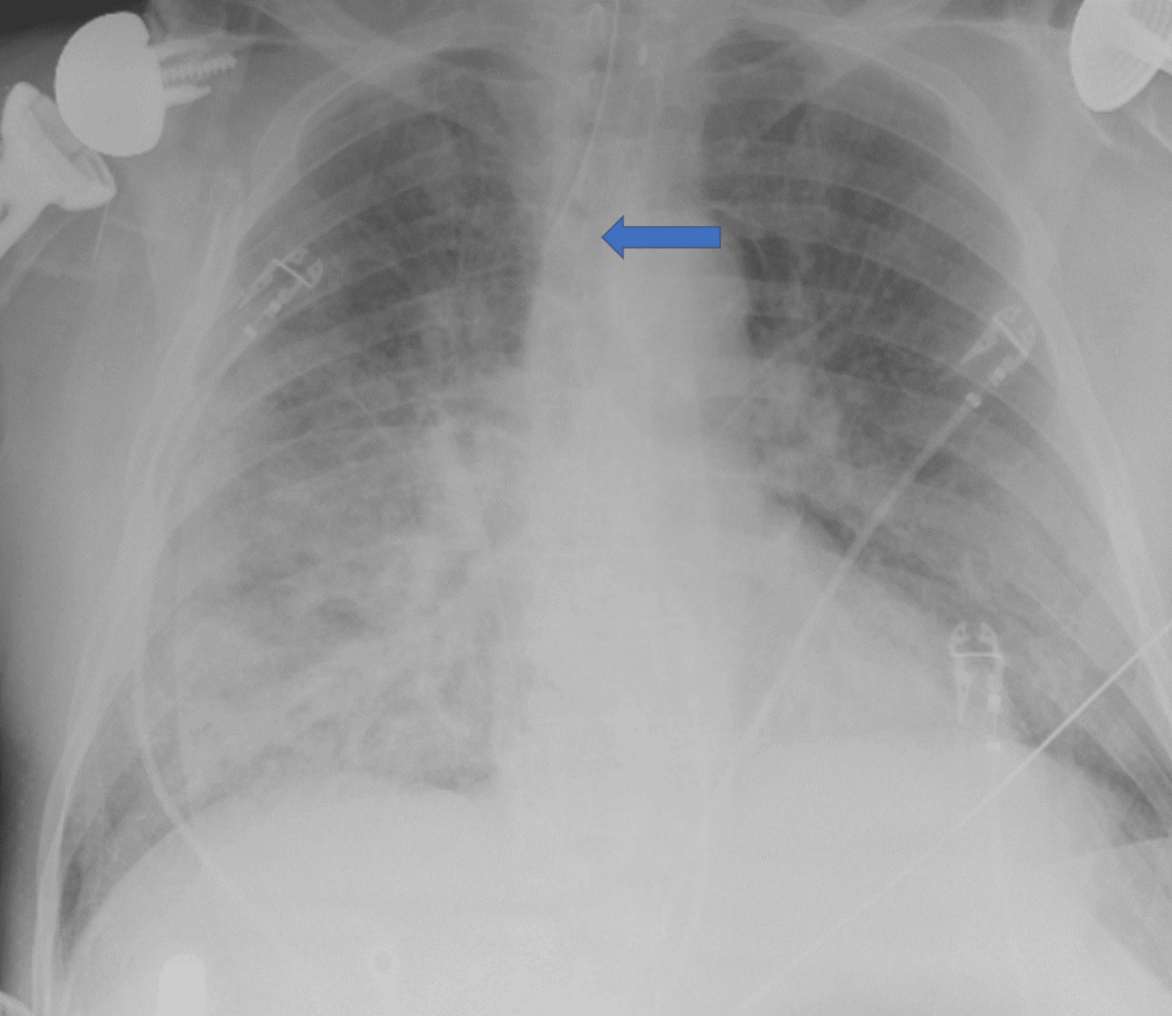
Post-intubation chest radiograph confirming endotracheal tube positioning
